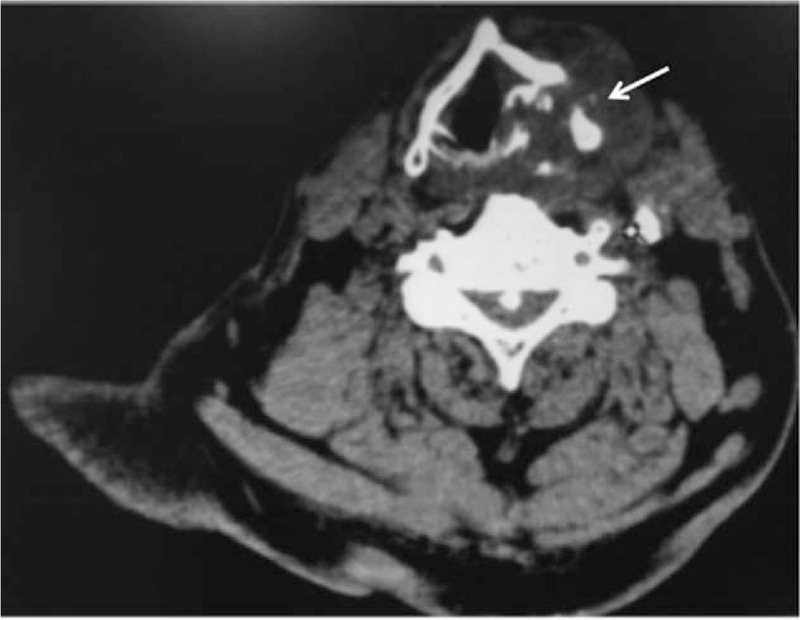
The CT of thyroid showed that the right thyroid lump and multiple calcifications, the thyroid cartilage was compressed and became deformed and reached the right subglottic region. CT = computed tomography.
